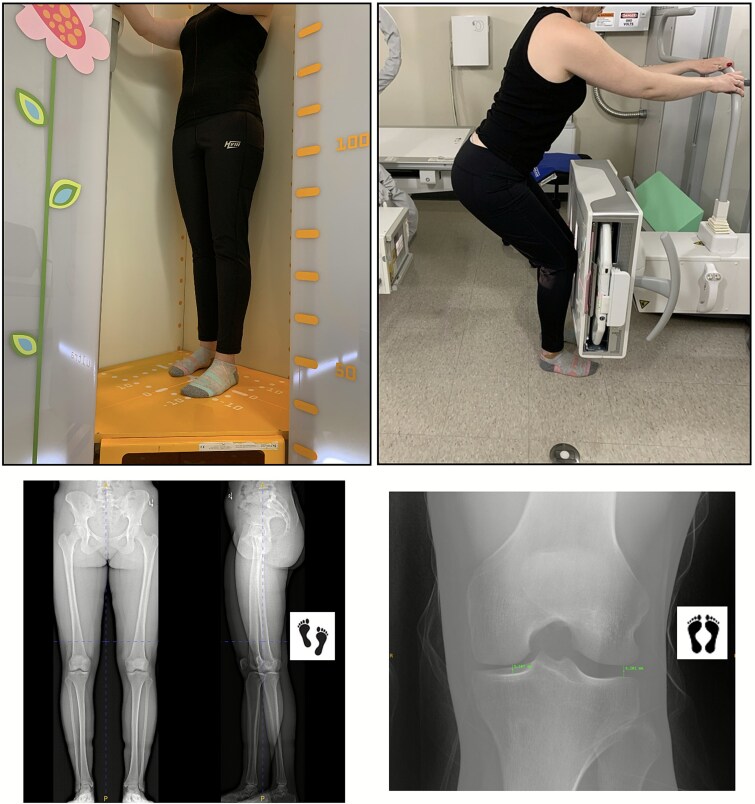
Anterior offset left foot placement for bi-planar lower limb radiographs (EOS Imaging) shown on left, with images below. Conventional radiography had both feet positioned side by side, with a resulting example image shown below.
